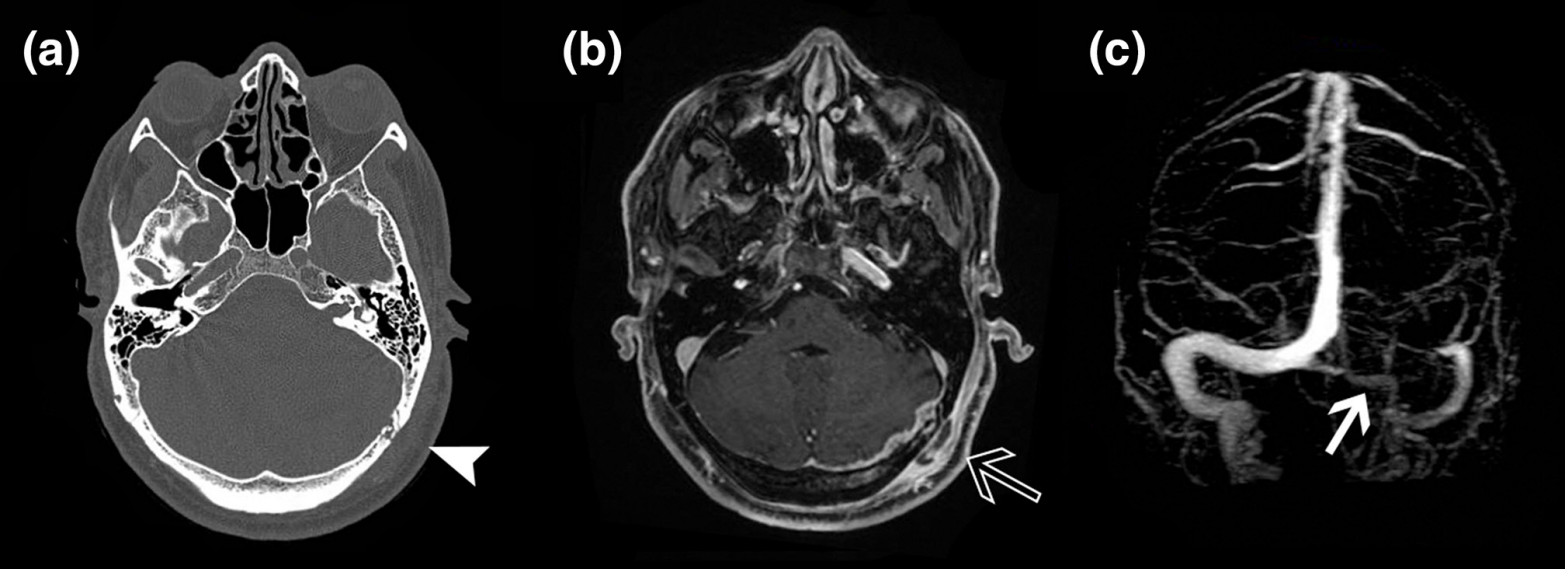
(a) The axial view of CT bone window of the skull showing bony erosion near the left TS and adjacent soft tissue swelling (white arrowhead). (b) The axial view of contrast MRI scan of the brain showing enhancement involving the dura, occipital bone and overlying scalp (empty arrow). (c) The coronal MIP reformation of MR venogram of the brain showing Lt TS thrombosis (white arrow).
